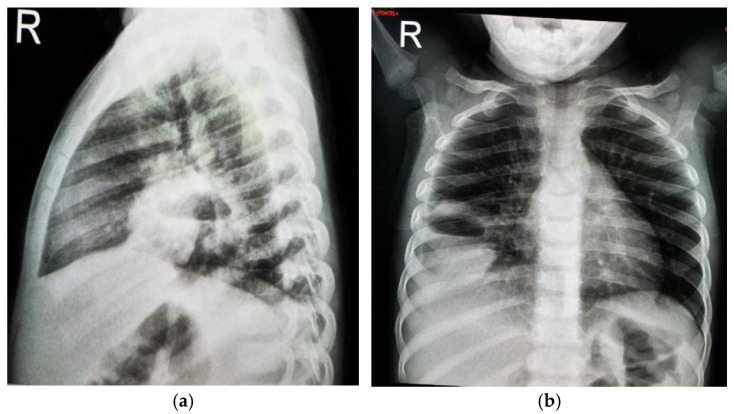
Two-year old male child with pulmonary abscess: oval mass with air–fluid level and blurred margins in right lower lobe; (a) lateral chest X-ray; (b) posteroanterior chest X-ray.
